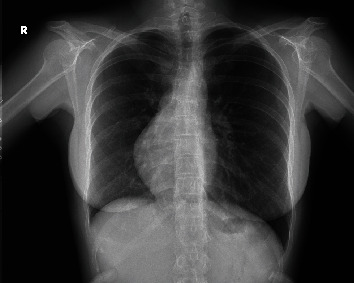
Chest X-ray showing the rightward shift of the heart.
